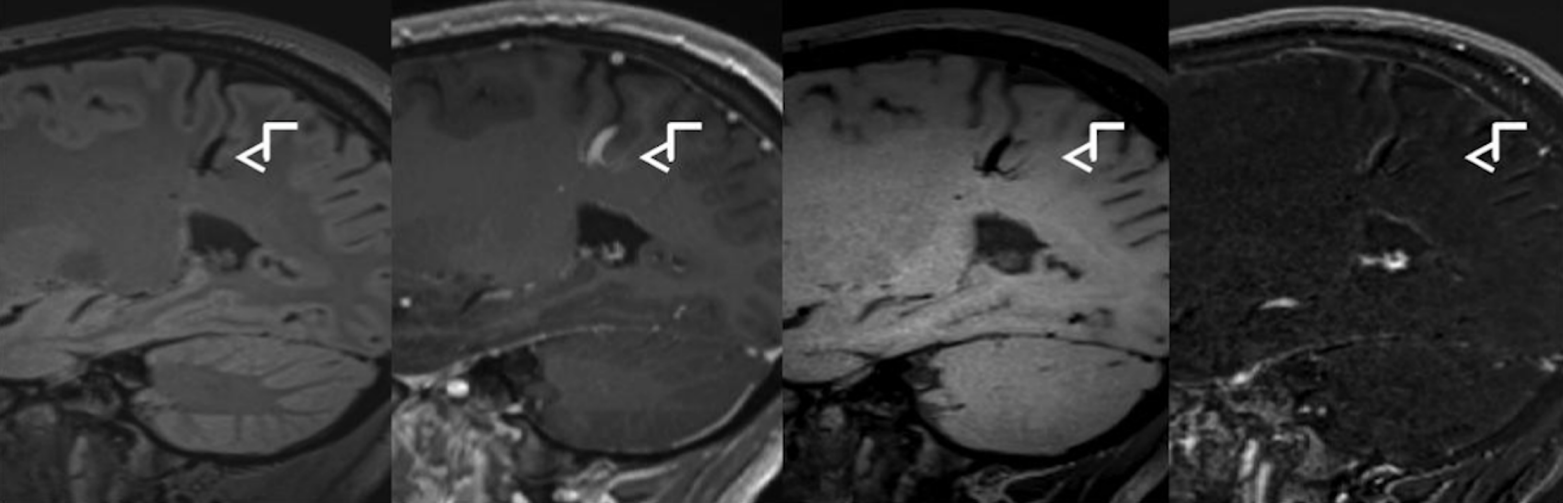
Suppression of vessel in T1w dark blood image. From left to right: FLAIR, T1w-MPRAGE after Gadolinium injection, T1w dark blood after Gadolinium injection, subtraction image.
